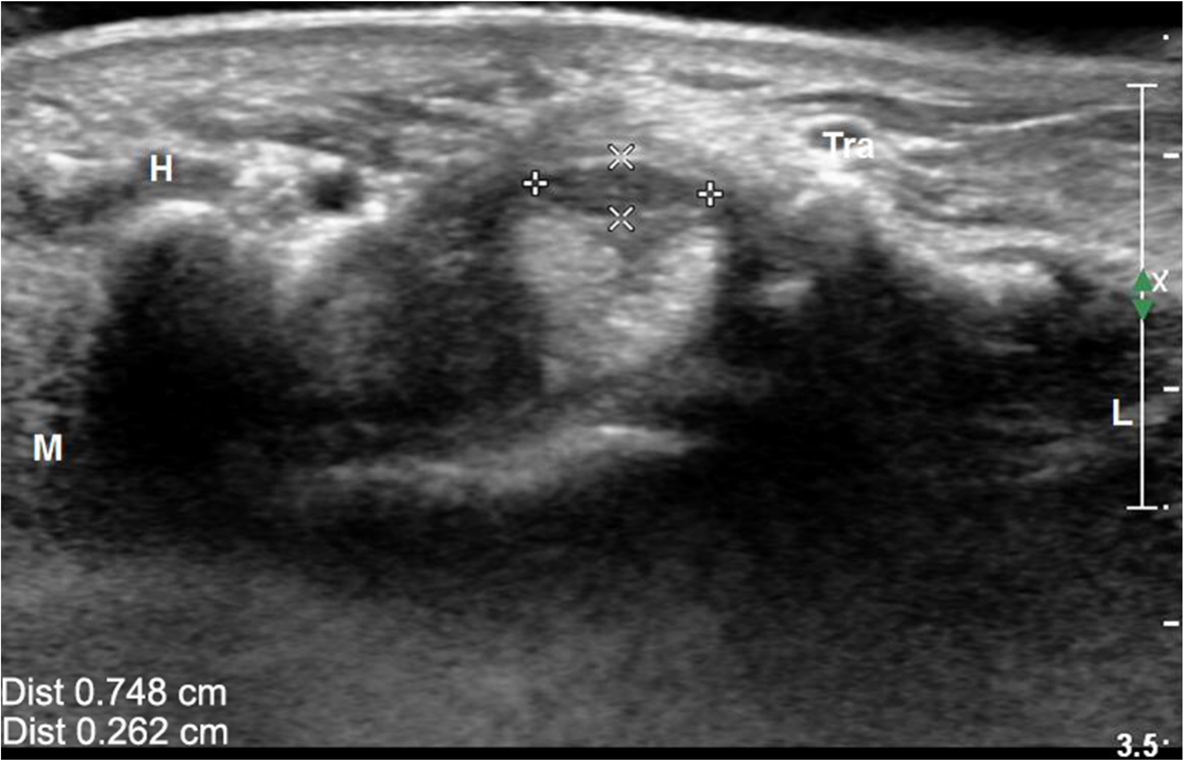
Transverse ultrasonic image of the distal carpal tunnel at the level of the pisiform bone showed median nerve compression. The gap between “++” suggests the left-right diameter of the median nerve. The gap between “xx” suggests the anteroposterior diameter of the median nerve. The left-right diameter and anteroposterior diameter of the median nerve at the level of the hamate bone was 0.748 and 0.262 cm, respectively. *H* hamate bone; *Tra* trapezium bone; *L* lateral; *M* medial
